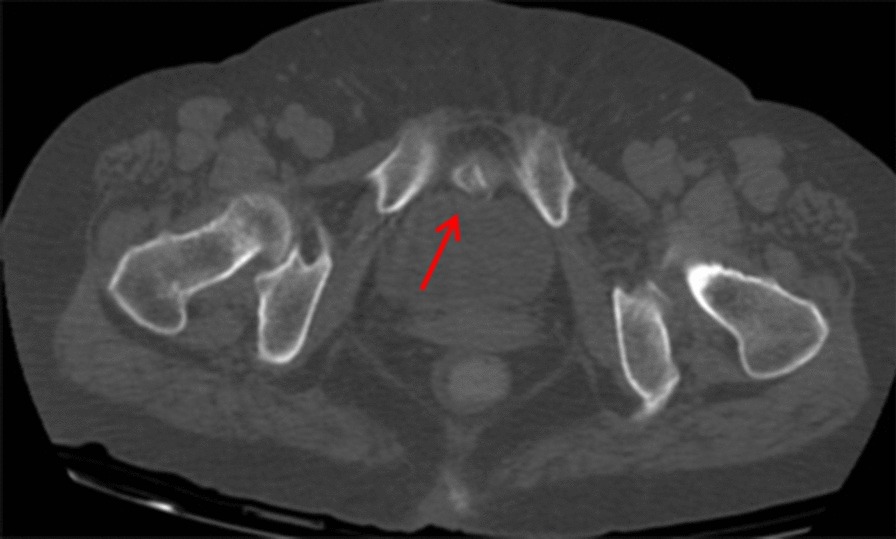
Primary abdominal CT showed a high-density mass in front of the bladder that compressed the antetheca (arrow)
